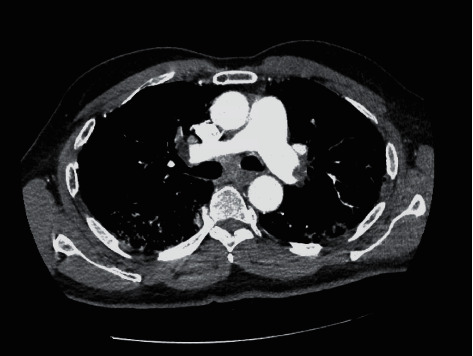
CT angiogram showing pulmonary emboli in the distal right and left pulmonary arteries.
